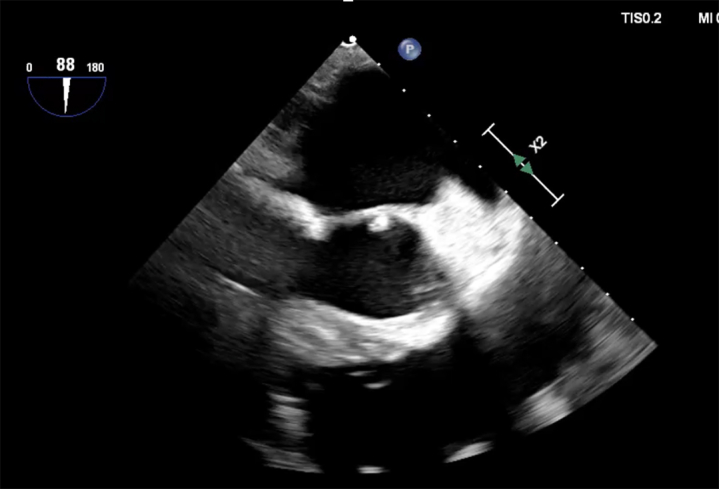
Echocardiographic demonstration of the mitral valve vegetation.
